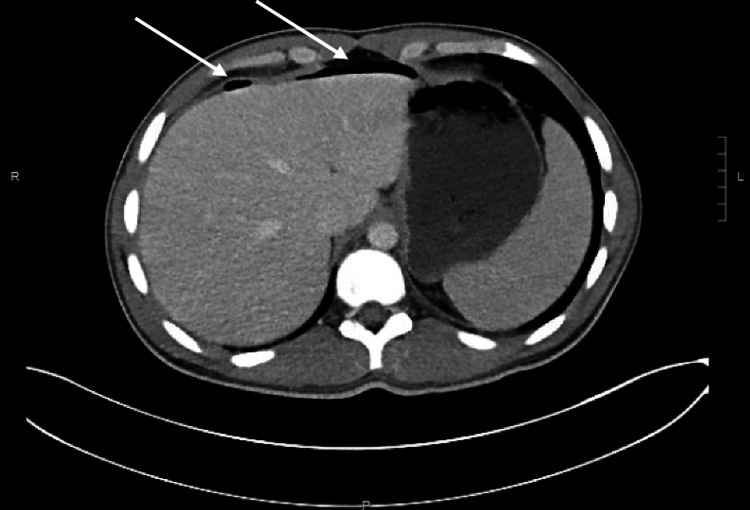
Pneumoperitoneum on CT imaging Arrows indicating pneumoperitoneum. CT: Computed tomography
